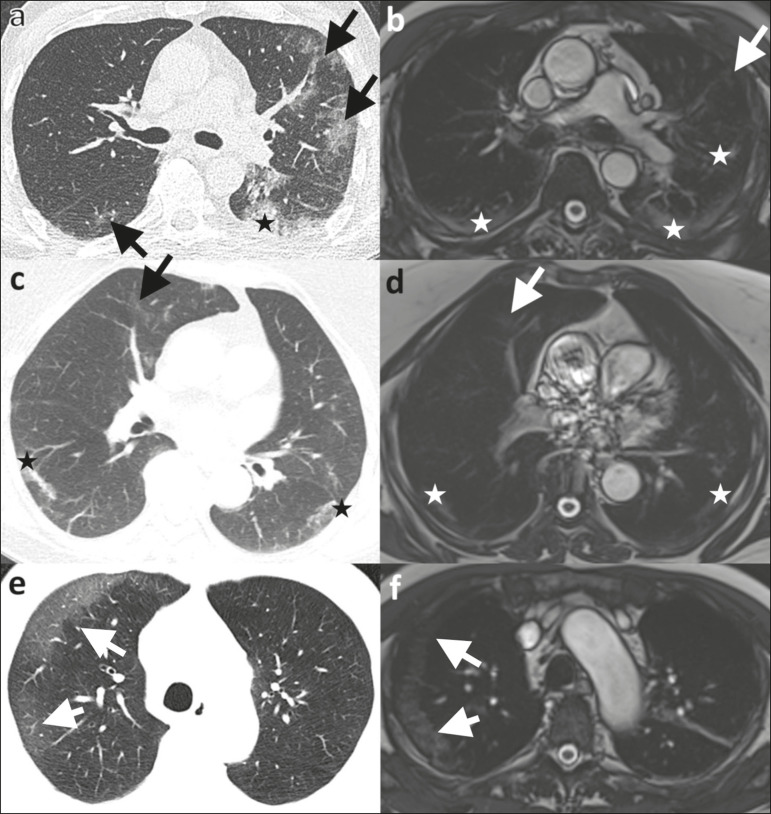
Ability of 1.5 T TrueFISP MRI to detect consolidations and ground-glass opacities. CT scan (a) showing peripheral ground-glass opacities of the left upper and right lower lobe (arrows), as well as consolidations of the left lower lobe (star), in a patient who was diagnosed with COVID-19 via RT-PCR two days later. MRI of the chest (b), performed 6 days after CT, showing consolidations in the lower lobes and left upper lobe (stars), with barely visible ground-glass opacities (arrow). Chest CT (c) of another patient, performed 14 days after confirmation of COVID-19 by RT-PCR, showing ground-glass opacities (arrow) and consolidations (stars), which are almost equally as visible on an MRI scan (d) acquired 2 days earlier. In another patient with COVID-19, ground-glass opacities with subpleural sparing in the right upper lobe (arrows) are present on a CT scan (e) performed on the day of COVID-19 confirmation by RT- PCR, as well as on an MRI scan (f) acquired 4 days later.
